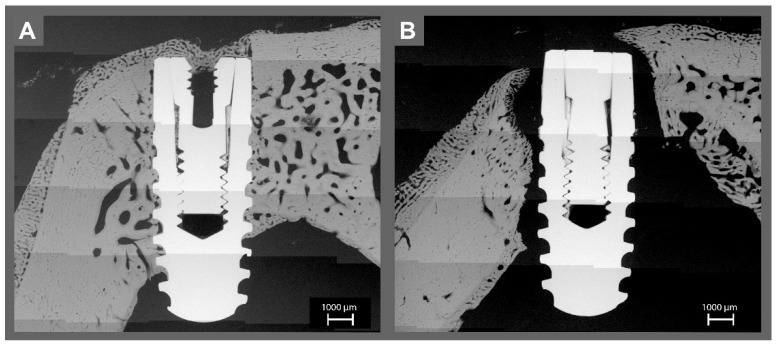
The micro-radiographic examination of the corresponding (A) sterile and (B) contaminated implants placed into the frontal bone. Please note the lack of osseointegration around the contaminated implant.
